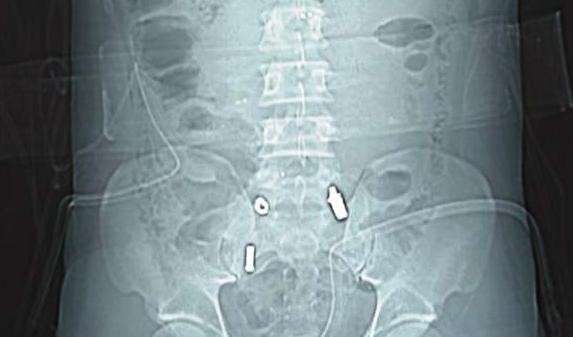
CT scan of our patient with bullets.
